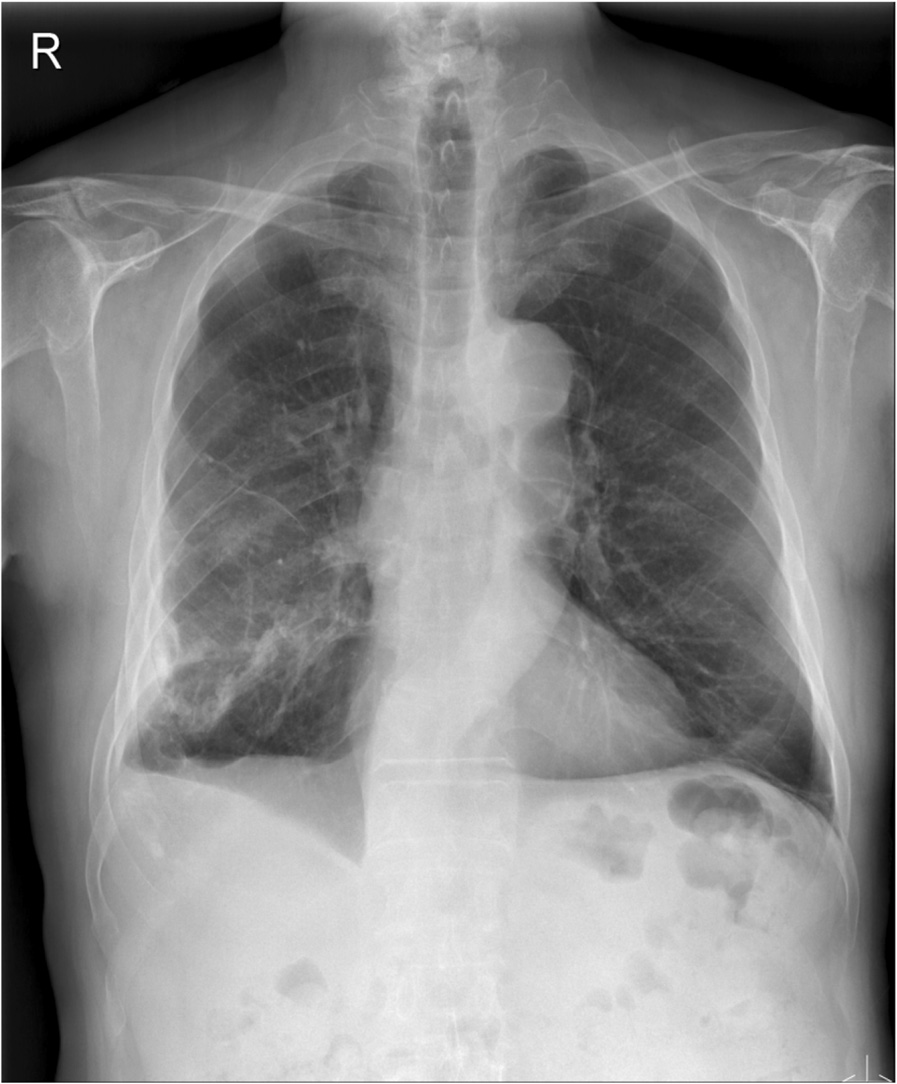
Plain chest radiograph indicates lucent upper lobes in both lungs suggesting emphysema. Evidence of lucent upper lobes in both lungs suggests emphysema. In addition, diffuse pleural thickenings with pleural calcifications present in the right middle and lower thorax. Linear atelectasis or fibrotic scars also present in the left lower lobe. Although the patient stated no history of asbestos exposure, asbestos exposure might have occurred while installing boilers.
